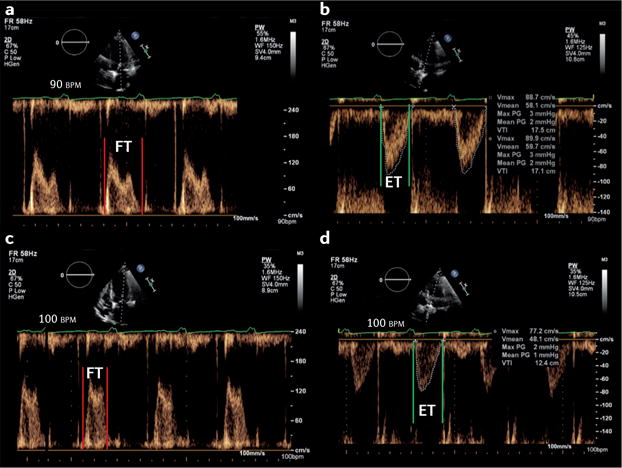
Echocardiography-guided cardiac output optimization using pulsed-wave Doppler imaging a,b | Transmitral and transaortic pulsed-wave Doppler imaging at 90 bpm. c,d | Transmitral and transaortic pulsed-wave Doppler imaging at 100 bpm. The filling time (FT) is measured from the start to the end of transmitral filling, and the ejection time (ET) from the start to the end of aortic ejection. The total ejection (t–ET) and filling (t–FT) periods are then derived as the product of the corresponding time interval and heart rate, and expressed in s/min. t–IVT (also in s/min) is calculated as 60–(t–FT + t–ET). A heart rate reduction of 10 bpm resulted in a reduction of t–IVT from 16.8 s/min to 10.0 s/min, and a corresponding increase in cardiac output from 3.6 l/min to 5.6 l/min.
